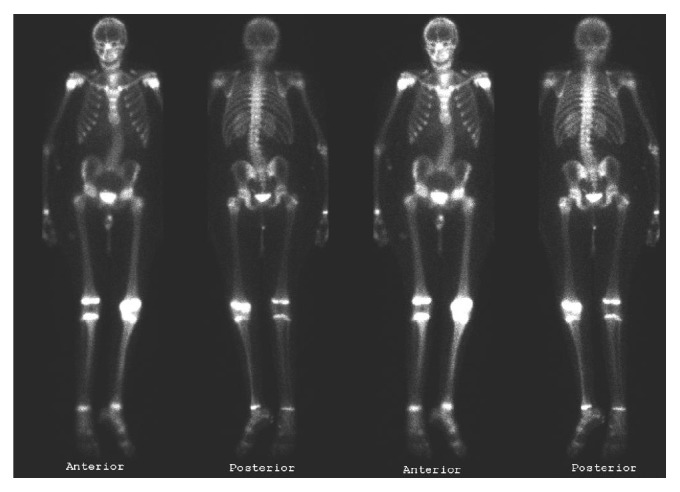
Three-phase bone scintigraphy, August 2009. Increased uptake in the distal left femur on delayed images.
